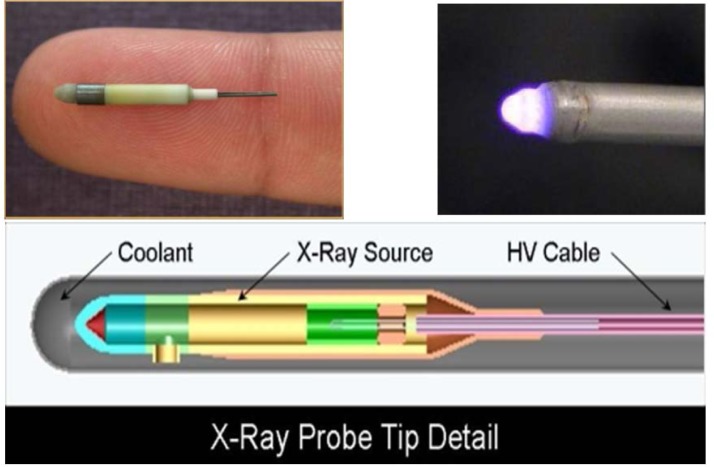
The Xoft Axxent miniature x-ray source.
